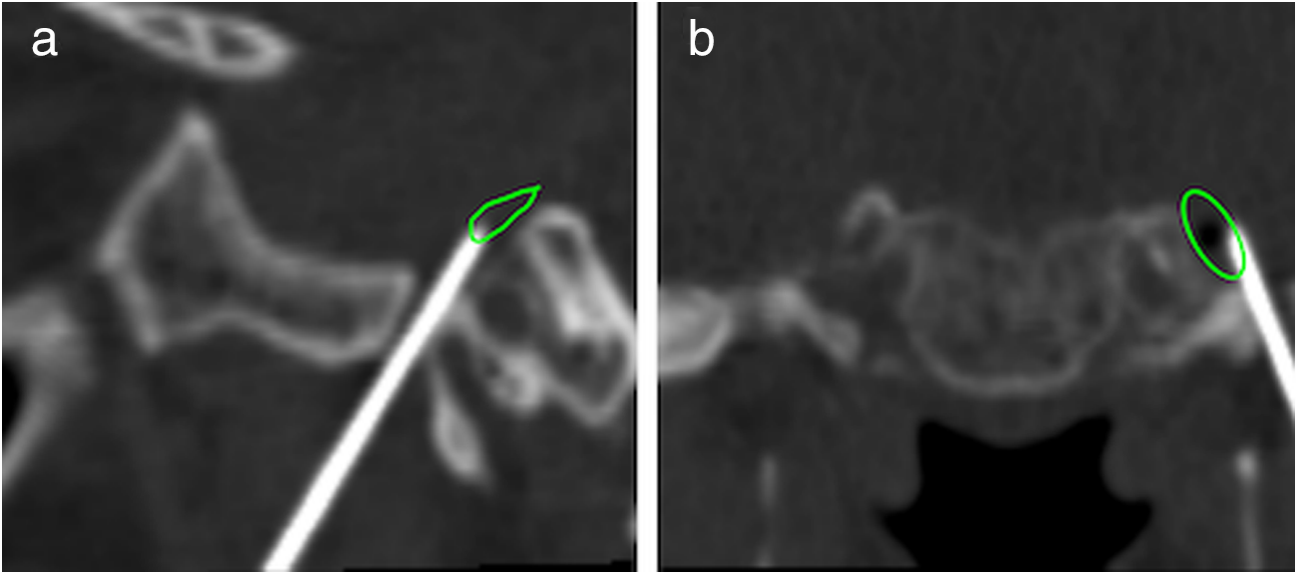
Computed tomography scan confirms the position of needle is adequate. Inline sagittal view (a) and inline coronary view (b)
